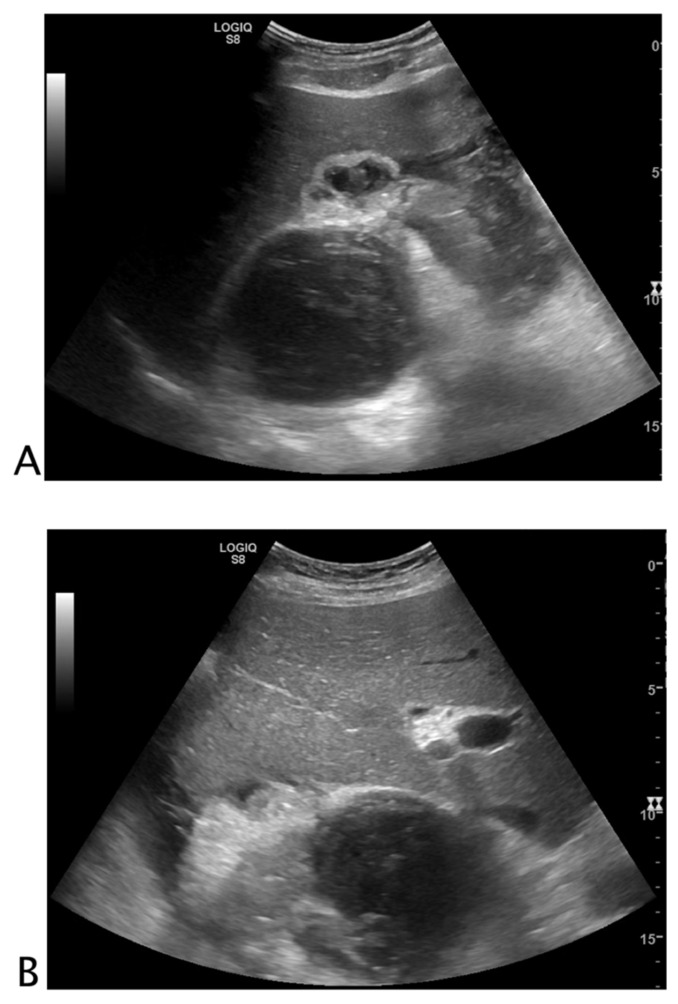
Black where it should not be. A 72-year-old male patient with abdominal pain and malaise after right nephrectomy. The right renal lodge was occupied by loculated fluid collection with fluid/fluid levels and clot (A). Perihepatic free fluid with inhomogeneous clot was detected (B). A suspected ultrasound diagnosis of post-surgical bleeding was formulated. The patient underwent CT angiography that confirmed the bleeding, and arterial endovascular embolization. Final diagnosis: post-nephrectomy bleeding.
